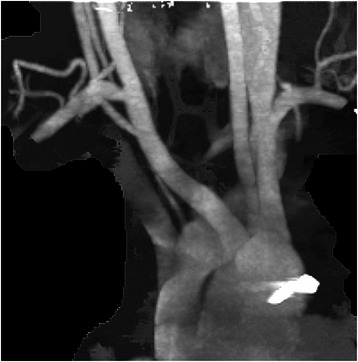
Follow up CT angiography showing complete ligation of the origin of ARSA and patent anastomosis of ARSA to right common carotid artery
